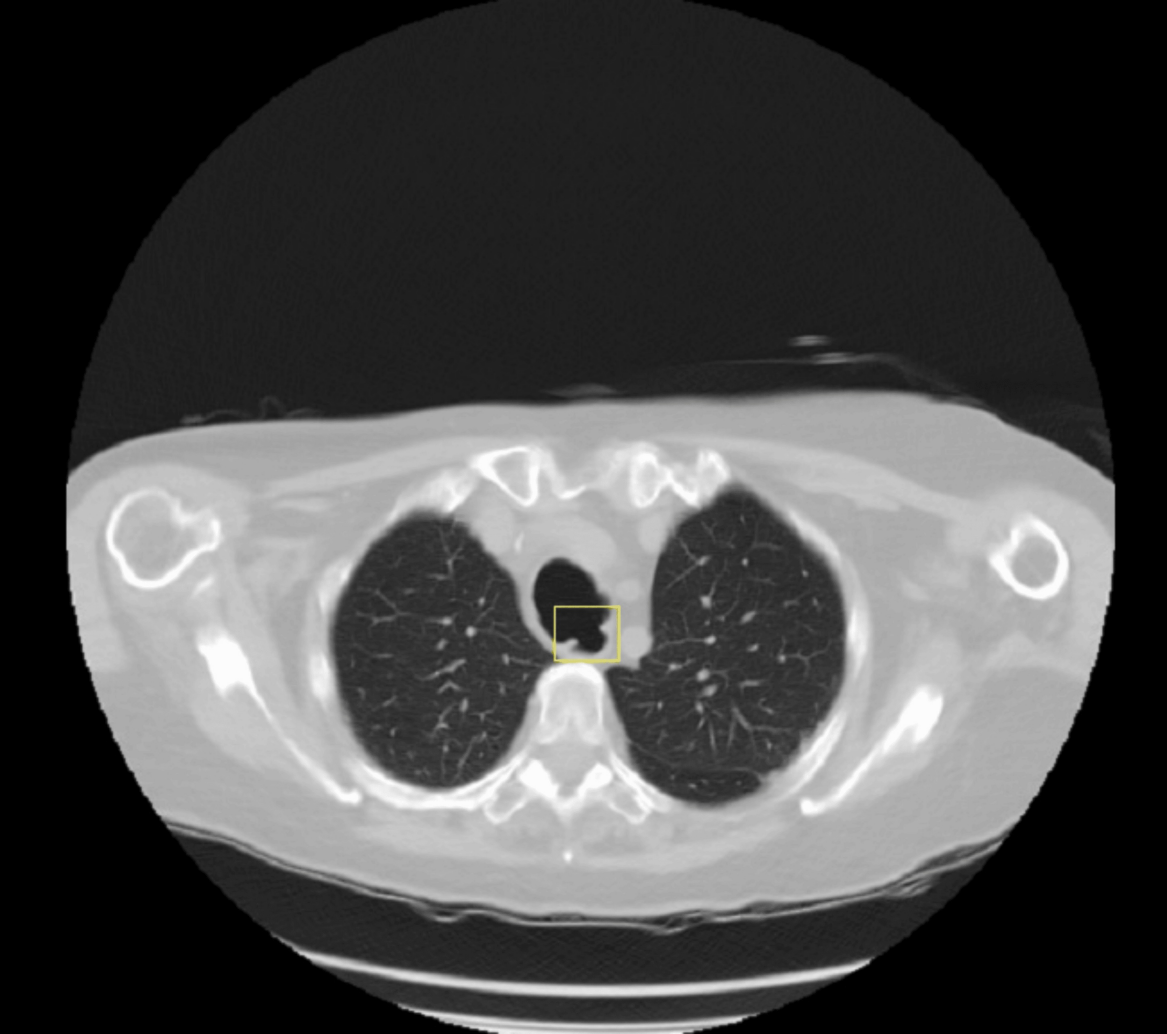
Axial view of non-enhanced CT Chest (Lung window) showing abnormal connection (yellow box) between the trachea (superior structure) and the esophagus (inferior structure), compatible with TEF TEF: tracheoesophageal fistula
